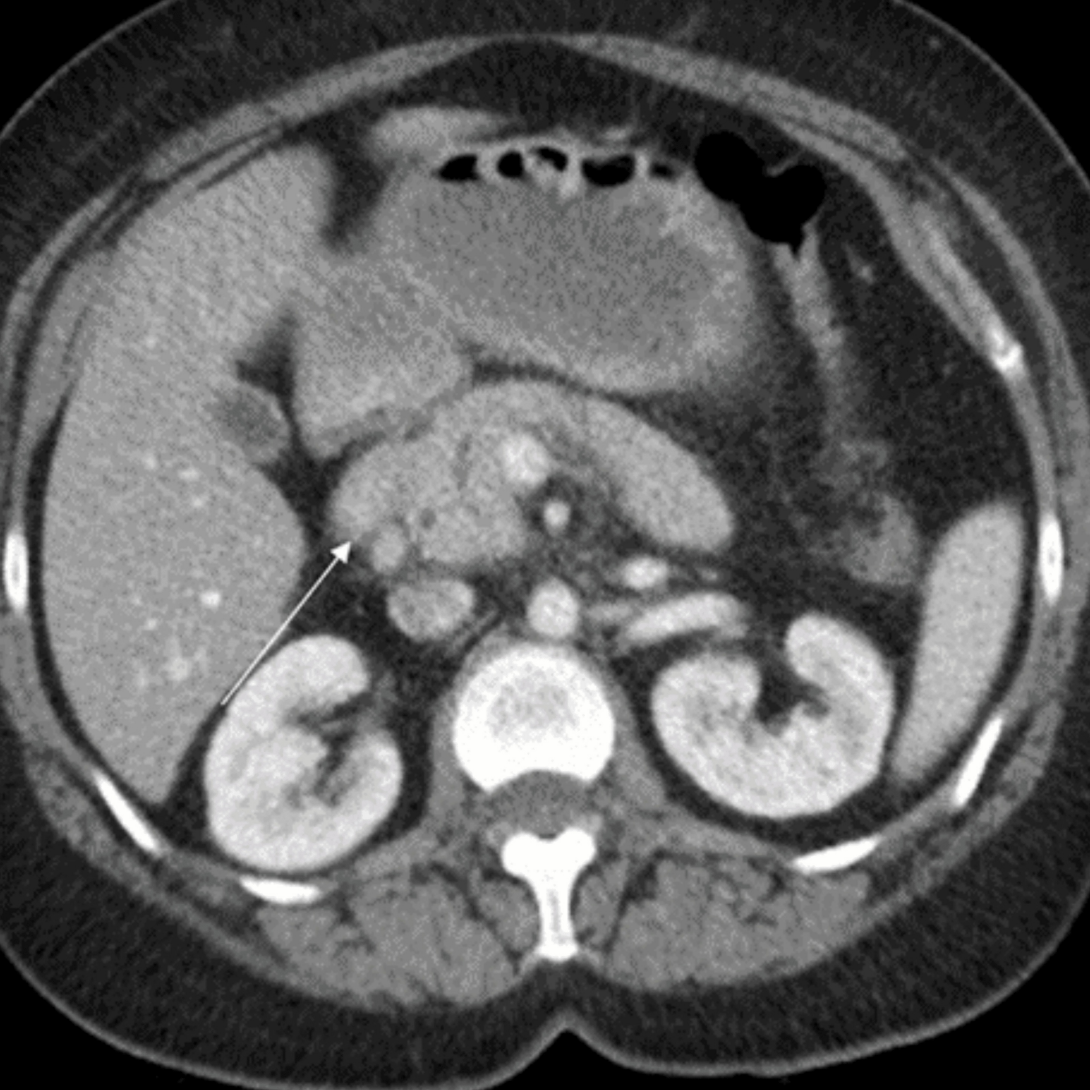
CT abdomen/pelvis showing mild acute interstitial edematous pancreatitis at the pancreatic head and uncinate process.
